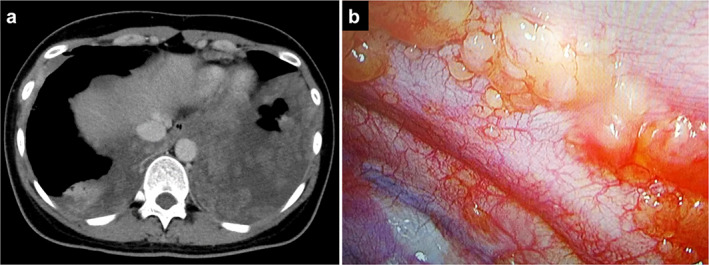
A patient with extraskeletal myxoid chondrosarcoma metastasizing to the pleura. (a) Chest computed tomography shows bilateral pleural effusions with multiple pleural nodules. (b) Thoracoscopic view shows multiple yellowish nodules with hypervascularity on the left parietal pleura
